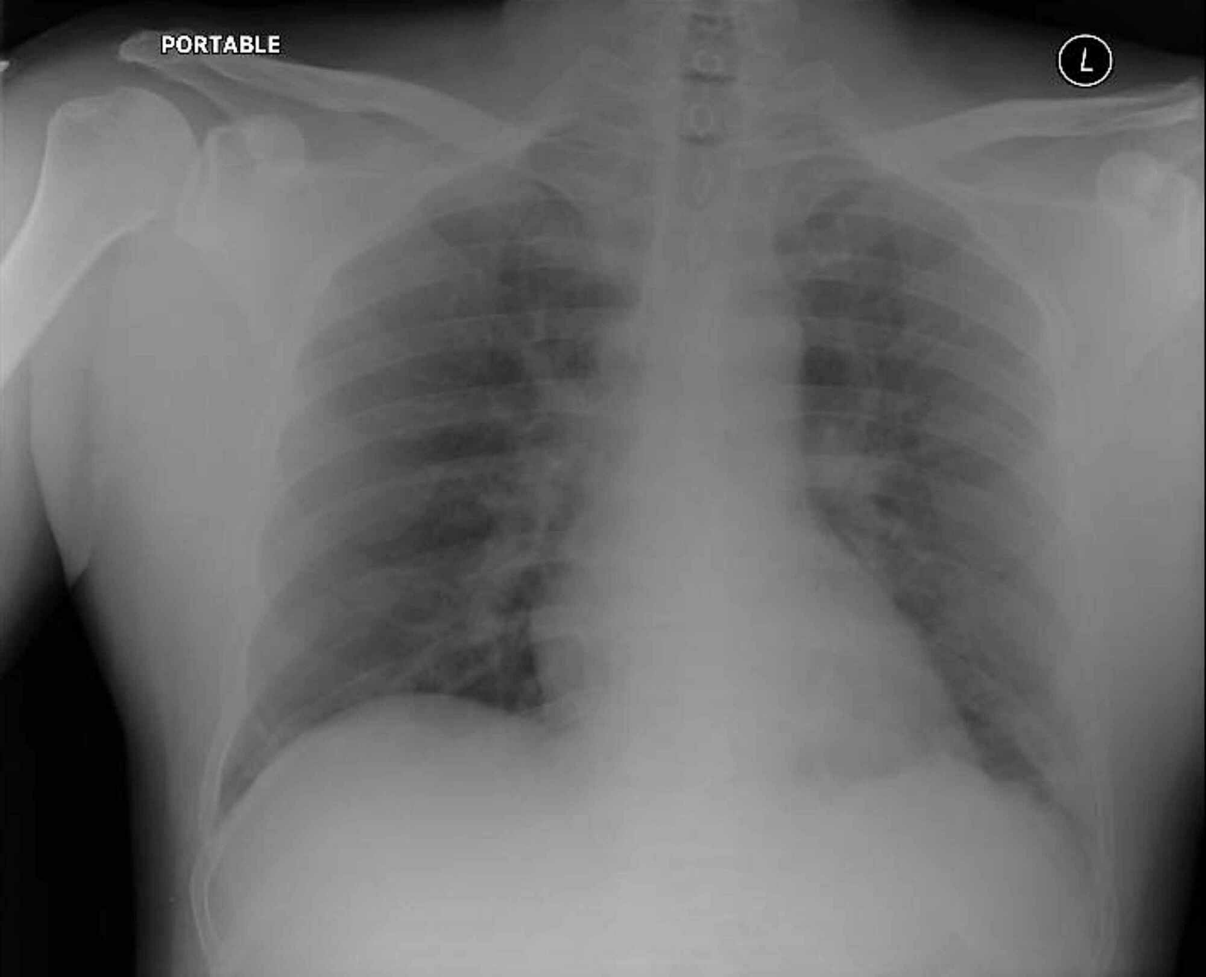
Chest X-ray following COVID-19 diagnosis. Chest plain radiography showing mild bilateral interstitial infiltrates.
